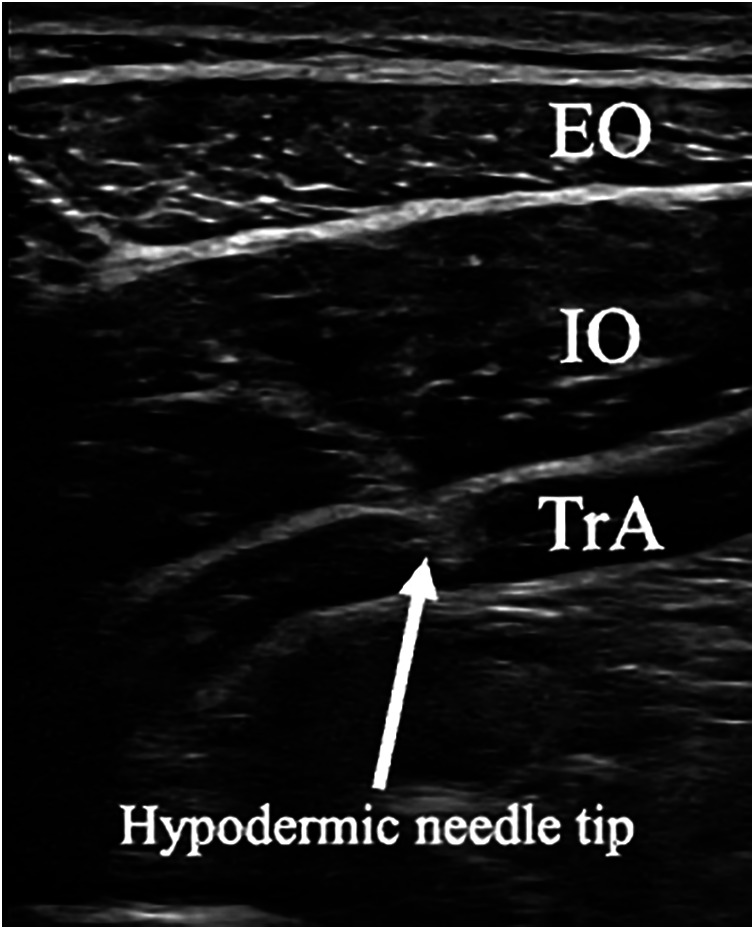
Ultrasound images of the hypodermic needles insertion to transversus abdominis (TrA). EO, external oblique; IO, internal oblique; TrA, transversus abdominis.
